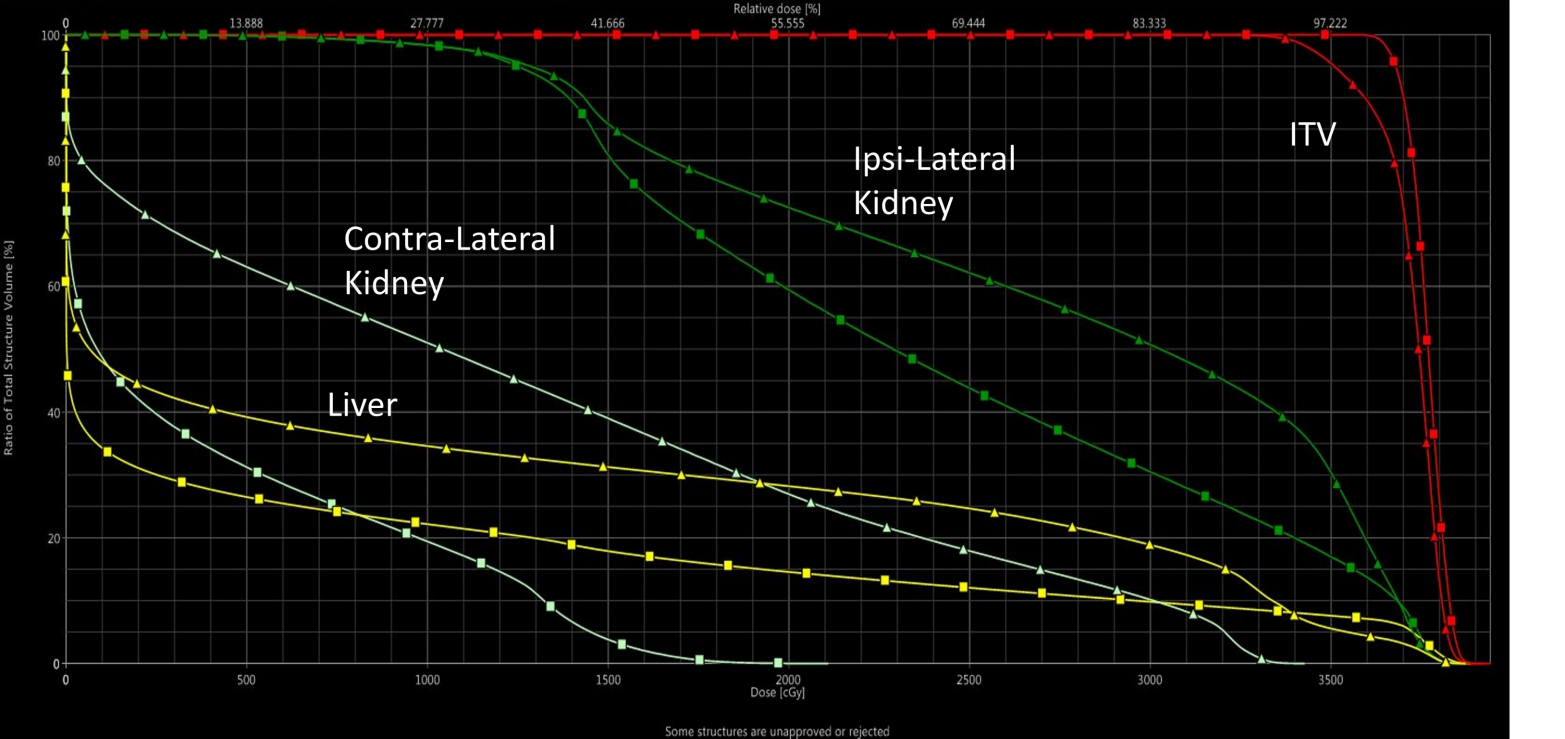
Impact of weight loss on treatment plan. (*squares*) initial treatment plan created and calculated on planning CT, (*triangles*) initial treatment plan re-calculated on a synthetic CT (i.e., planning CT deformed to anatomy of CBCT) x-axis: Dose (cGy); y-axis: Total Structure Volume (%) ITV: internal target volume, CT: computed tomogrpahy, CBCT: cone-beam computed tomography
